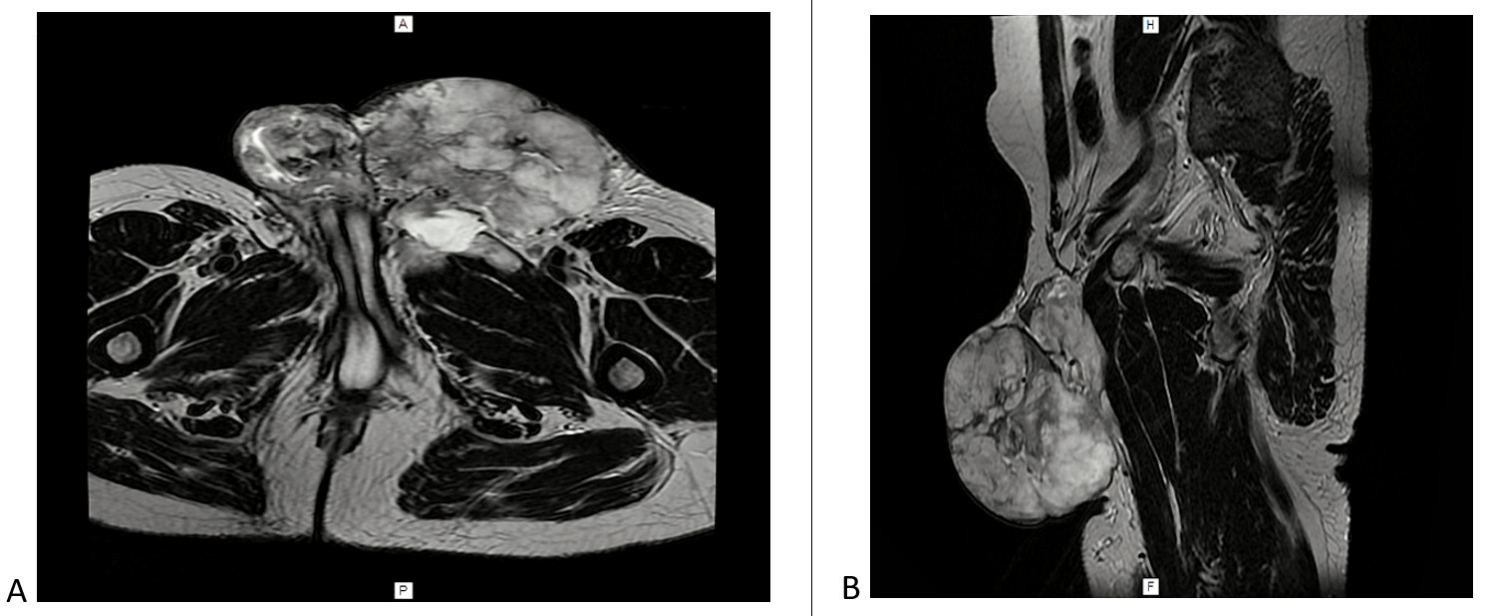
Magnetic resonance imaging. A) Cross section revealing inseparable inguinal mass with structures of the scrotum.
